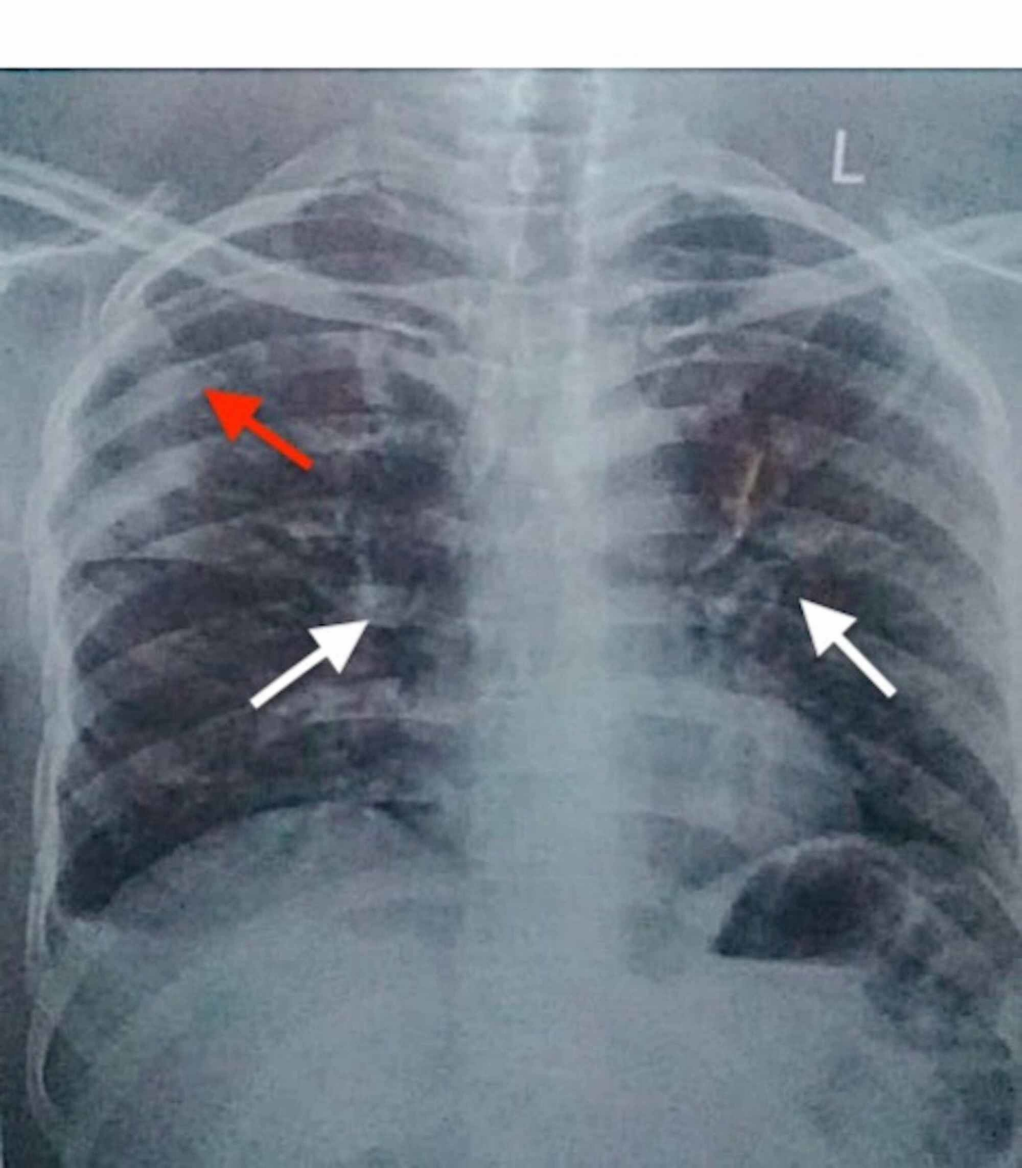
Chest radiograph showing pneumonitis patch in the peripheral region of right mid zone (red arrow) and bilateral alveolar infiltrates (white arrows).
